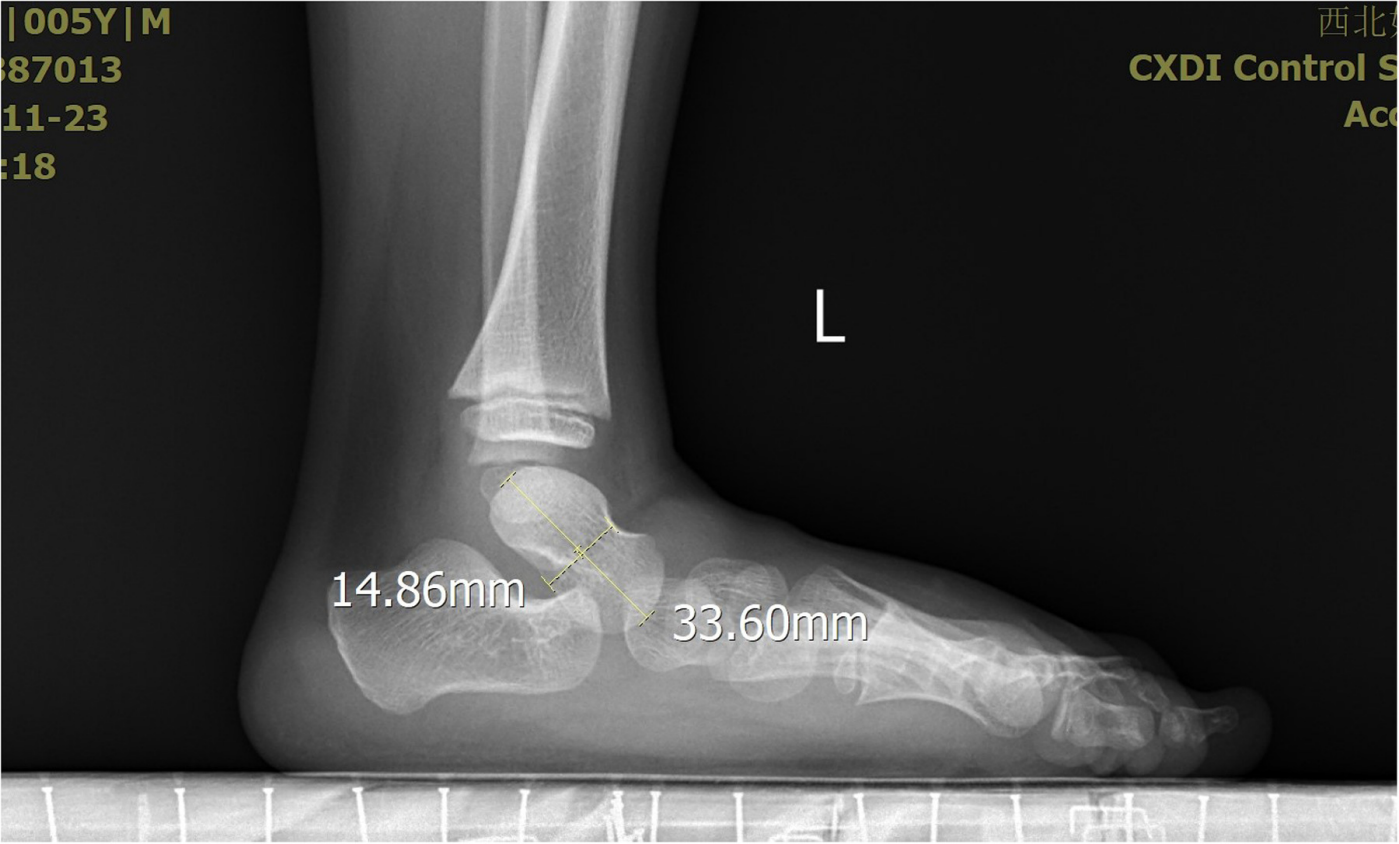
The X-ray demonstrated the measurement of the talus length and height.
